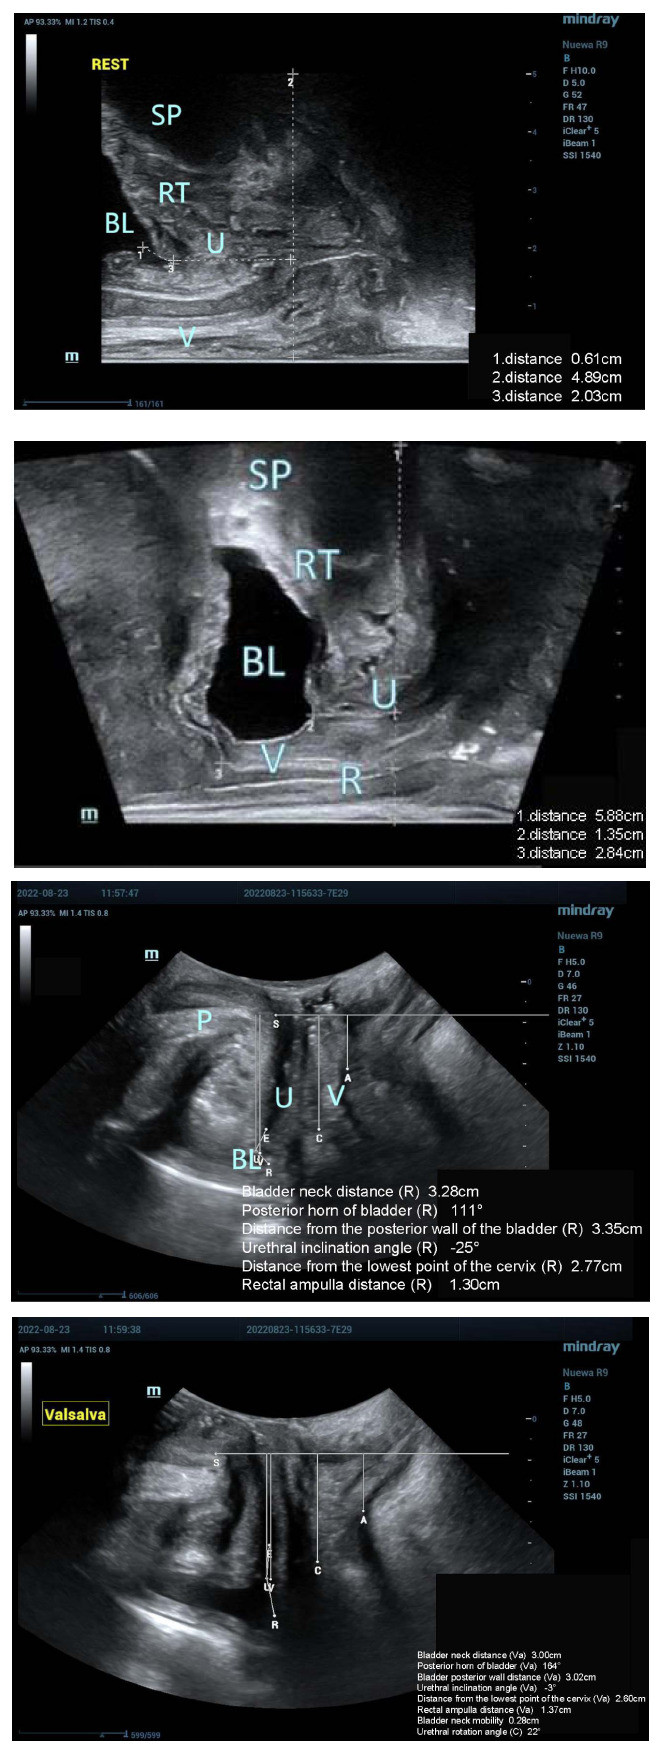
Ultrasound images of the study participants without SUI using the two ultrasound methods.
